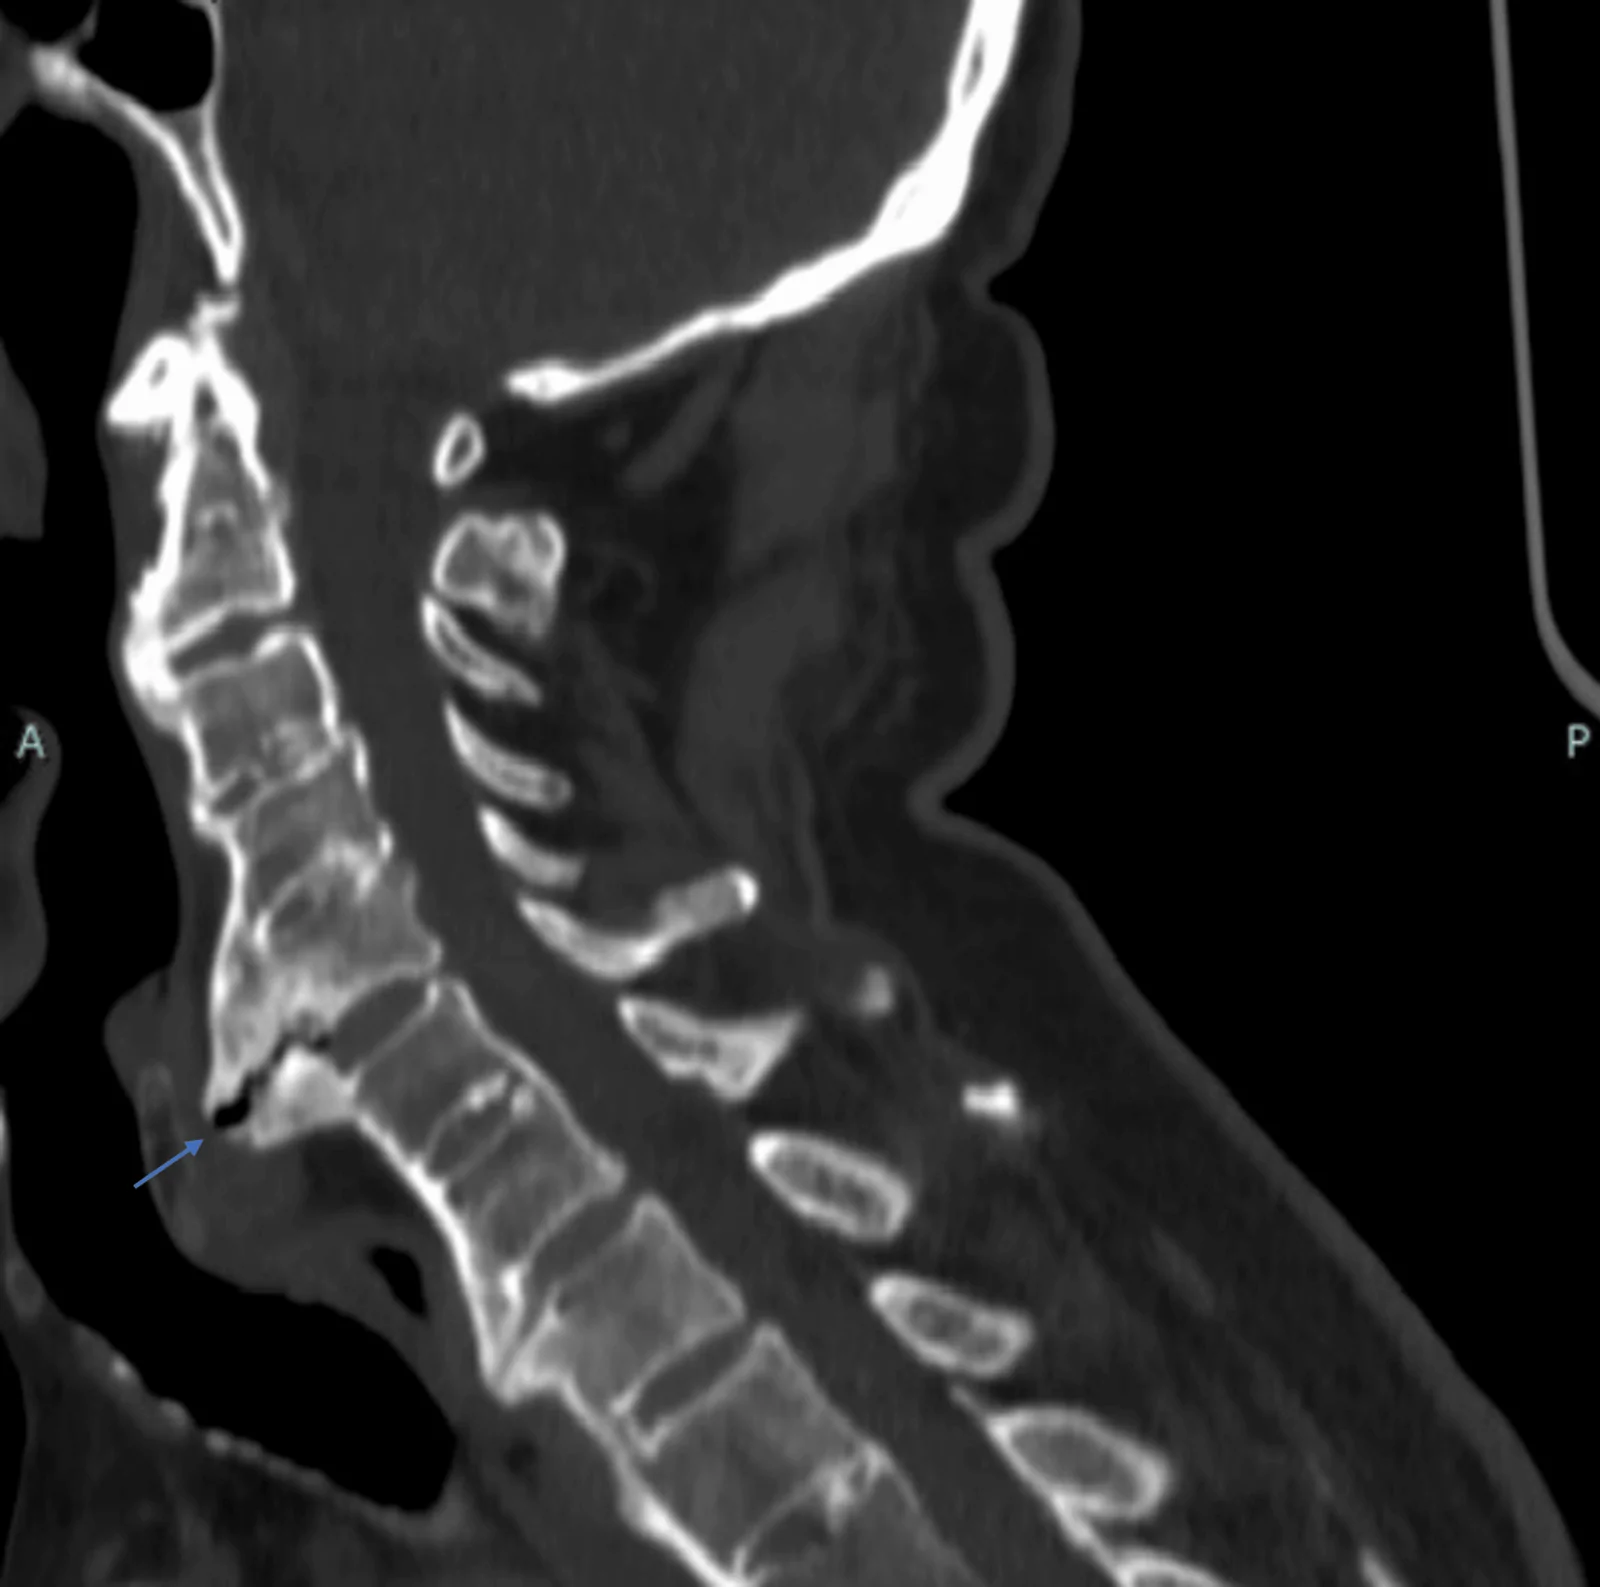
Computed Tomography (CT) Sagittal and axial cervical CT images showing prominent anterior marginal osteophytes, particularly at C5-C6, causing significant impression on the esophageal lumen (arrow). Findings are compatible with advanced diffuse idiopathic skeletal hyperostosis.
